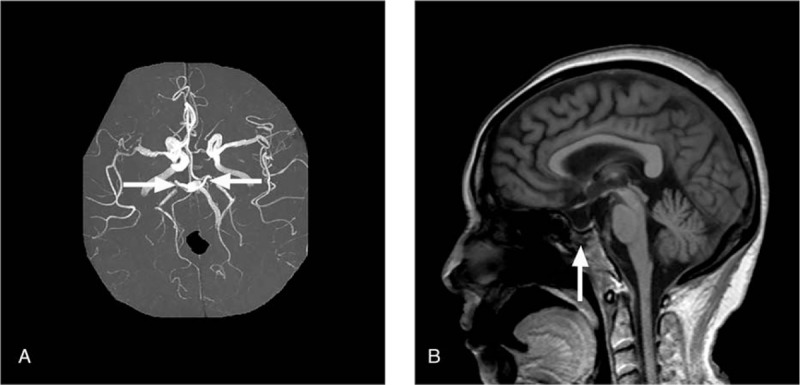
(A) Magnetic resonance angiography revealed whole brain cerebral atherosclerosis, especially bilateral posterior cerebral artery (arrows). (B) Sagittal T1-weighted image show the hypophysis has been injury severely (vacuole turcica) (arrow) and the nasal pharyngeal area is severely deformed.
